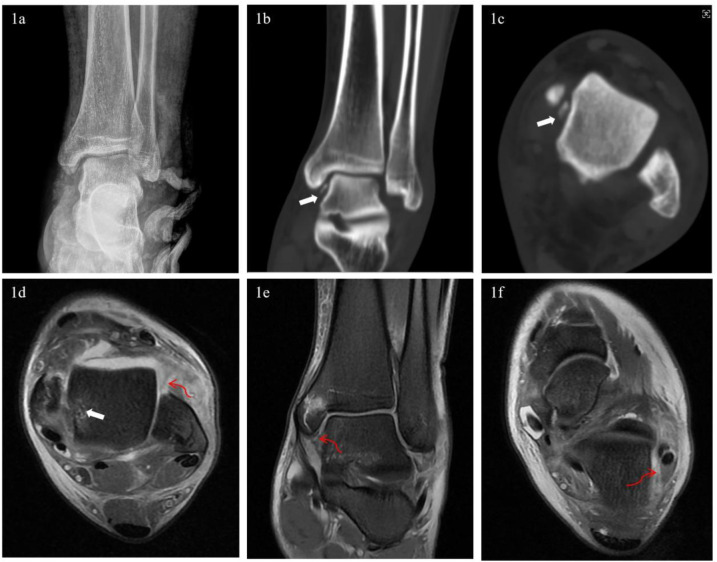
Preoperative imaging of the patient. (a) shows an anteroposterior x-ray of the left ankle with a fracture of the medial malleolus. (b) and (c) present coronal and axial CT images of the left ankle, highlighting a small bone fragment in the medial joint space. (d) displays an axial MRI of the left ankle, revealing bone marrow edema in the talus and rupture of the anterior talofibular ligament. (e) shows a coronal MRI of the left ankle, demonstrating a tear of the deltoid ligament, while (f) provides an axial MRI image depicting the rupture of the calcaneofibular ligament.
